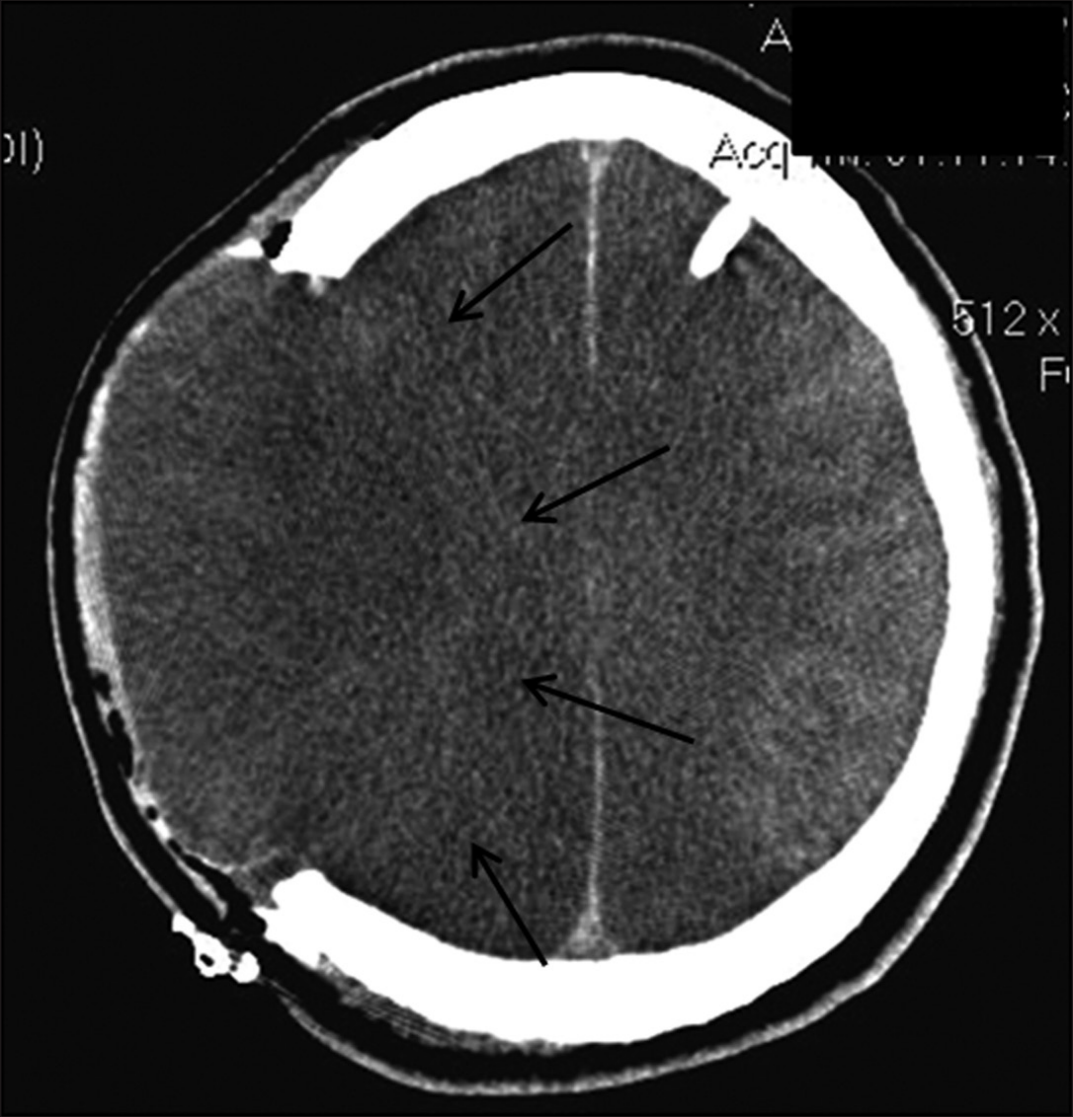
Axial CT scan showing total right hemispheric infarction, herniation of the brain through the defect, massive bihemispheric swelling, loss of white-gray matter differentiation
